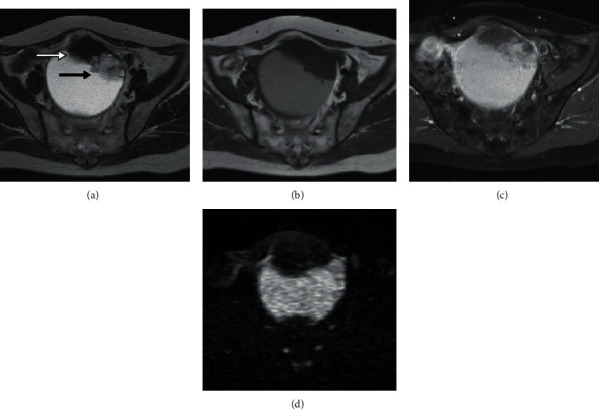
MR findings for the borderline Brenner tumor in a 54-year-old woman. (a) The axial T2WI shows a unilocular cystic tumor in the pelvic cavity which was 9 cm in diameter with two solid components. The cystic content has the same signal intensity (SI) as water. The solid mass located at the anterior wall (white arrow) shows low SI similar to the SI of muscle. The papillary tumor on the left side of the solid mass (black arrow) shows an intermediate SI, slightly higher than the SI of muscle. (b) The axial T1WI shows the cystic content has slightly higher SI than water. The solid mass shows slightly lower SI than muscle. The papillary tumor on the left side of the solid mass shows low SI, similar to the SI for muscle. (c) Gadolinium-enhanced fat-saturated T1WI shows the solid mass is slightly enhanced and the papillary tumor is well enhanced. (d) Axial DWI (*b* = 0, 1000 s/mm^2^) shows a solid mass with a low SI and the papillary tumor with high SI.
